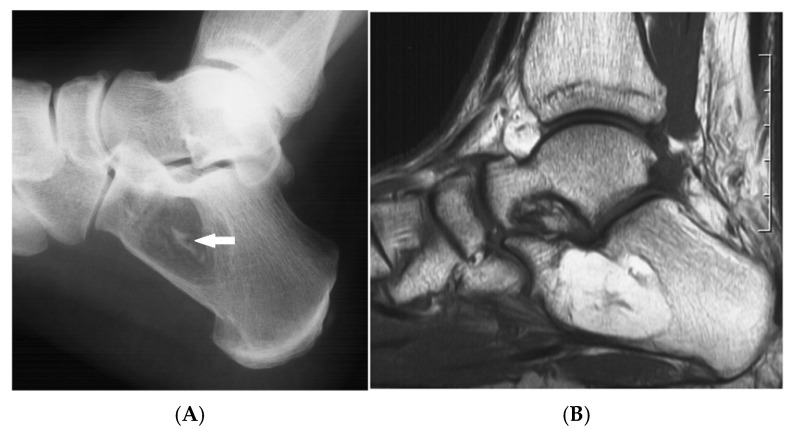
Intraosseous lipoma, calcaneum. (A) Plain radiograph demonstrating the classic location of the lesion with “bull’s eye” sign (solid white arrow). (B) Sagittal T1W MRI with fat-containing lesion.
